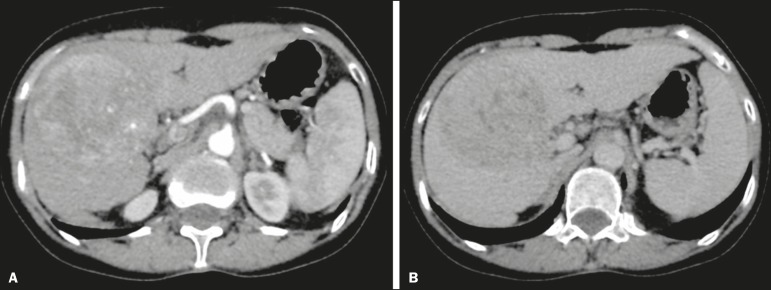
LI-RADS 5 observation. CT in the arterial and portal phases (A and B, respectively) showing a hypervascular observation > 20 mm (A), with washout (B), in a patient with hepatitis B.
